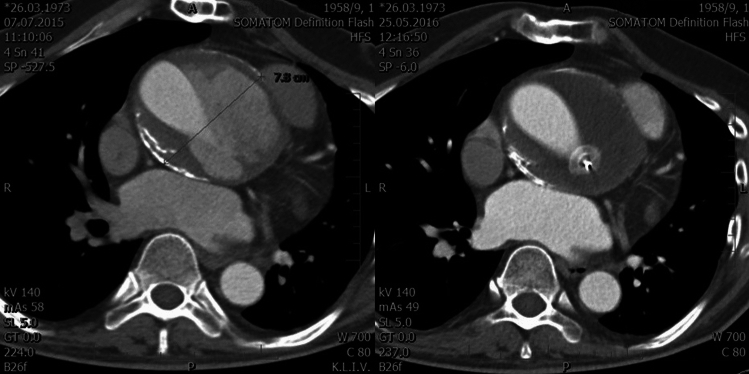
CT scan before and after occluder implantation
